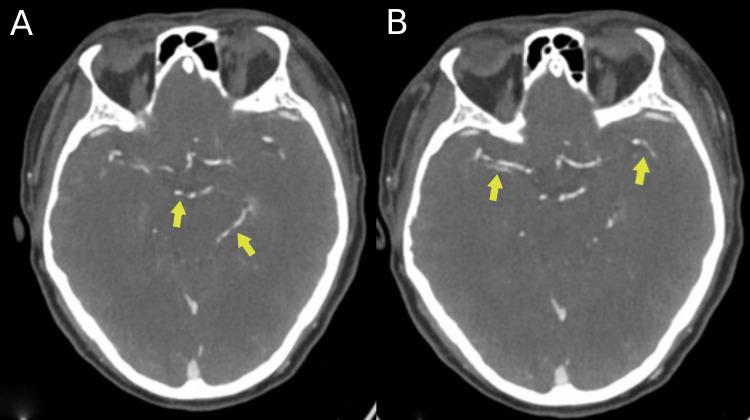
CT angiography of intracranial vessels CT angiogram reveals segmental beaded vascular irregularities, noted in the posterior cerebral arteries (A) and in the middle cerebral arteries, particularly in the M2 branches (B). These findings in our patient's clinical presentation and radiological findindgs were suggestive of vasculitis.
